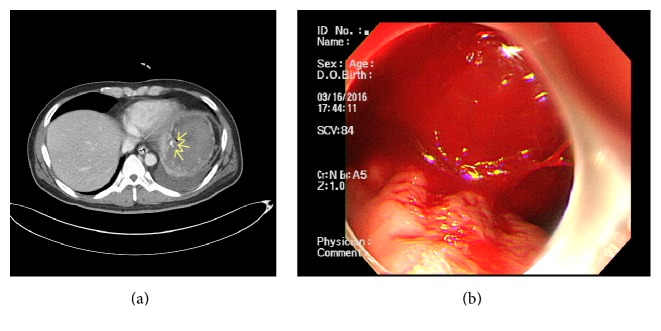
(a) An abdominal CT scan revealed bleeding gastric varices. (b) Esophagogastroduodenoscopy revealing bleeding from fundal gastric varices. Endoscopic hemostasis failed because of large clots and ongoing bleeding.
